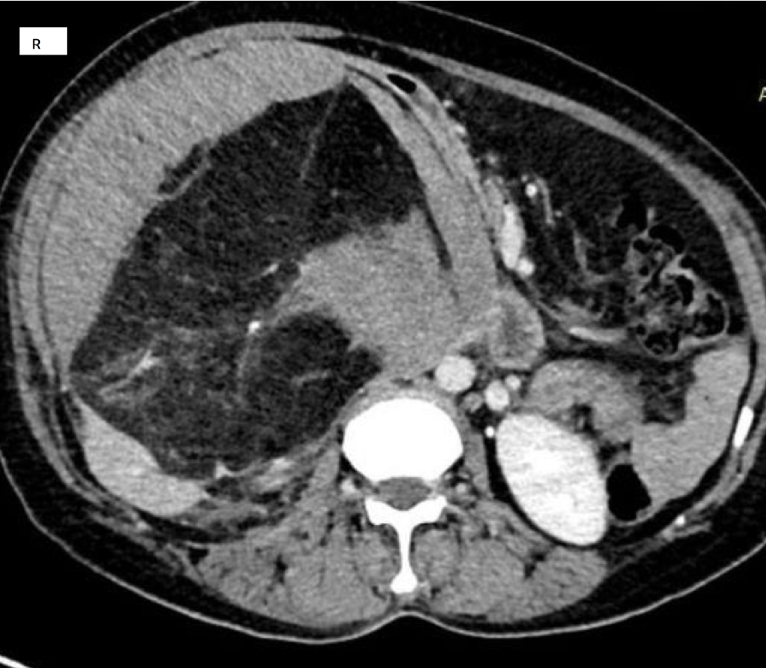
Abdominal CT scan, showing mixed density mass arising from the right kidney.
